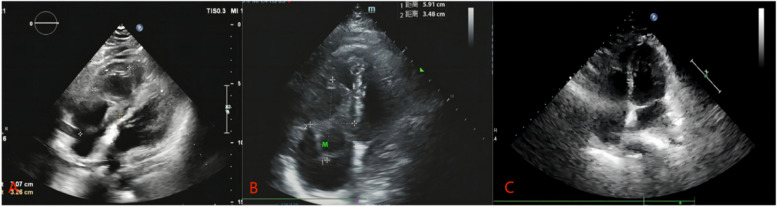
A-B Preoperative echocardiography images show the presence of the tumor. C Postoperative echocardiography image shows normal heart chambers without tumor
